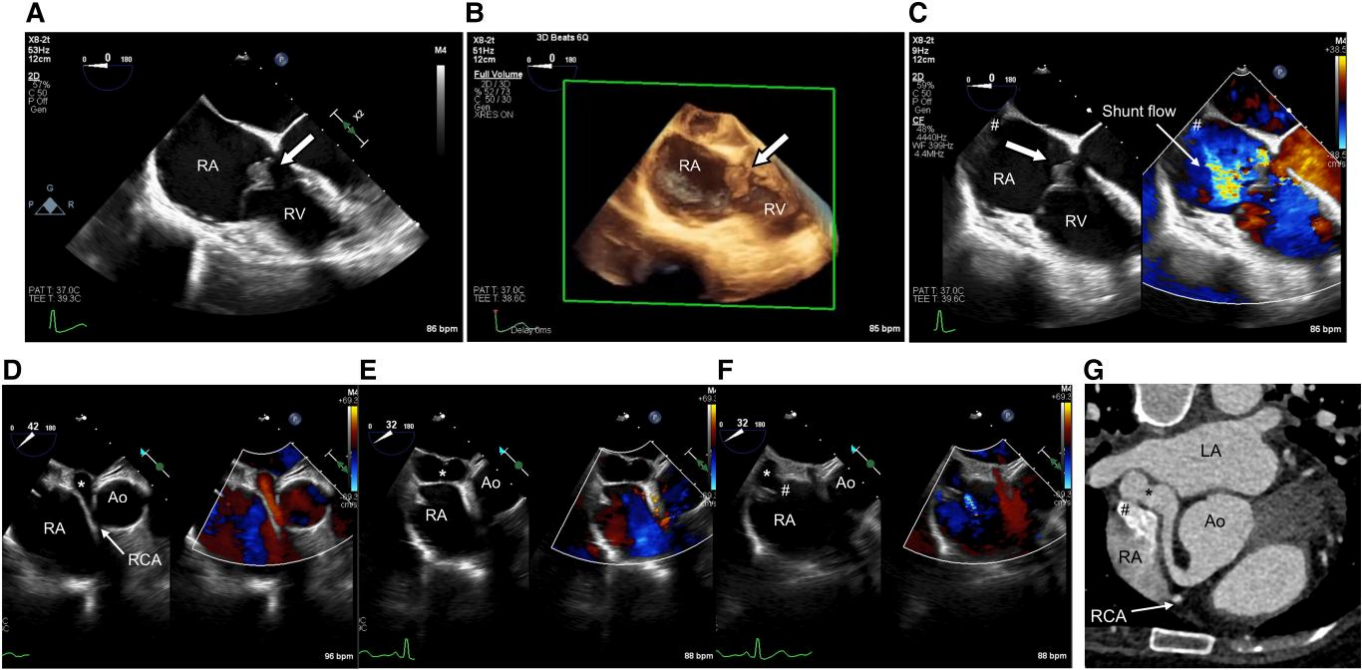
Transoesophageal echocardiography demonstrates vegetation attaching to the tricuspid valve and turbulent shunt flow from coronary artery fistula to the tricuspid valve. (*A, B*) Transoesophageal echocardiography and 3D transoesophageal echocardiography showed the vegetation attaching to the anterior leaflet of the tricuspid valve. (*C*) Transoesophageal echocardiography showed turbulent shunt flow towards the tricuspid valve from the outlet of the fistula to the RA (number sign). (*D*) Transoesophageal echocardiography showed coronary artery fistula (asterisk) originating from the right coronary artery, (*E*) running around the right atrium, and (*F*) terminating at the right atrium. The number sign shows the outlet of the fistula to the right atrium. (*G*) An axial oblique slice on computed tomography coronary angiography is flipped vertically and horizontally to align with the transoesophageal echocardiography image showing that the coronary artery fistula (asterisk) terminates at the right atrium. The number sign shows the outlet of the fistula to the right atrium. RA, right atrium; RV, right ventricle; Ao, aortic root; LA, left atrium; RCA, right coronary artery.
